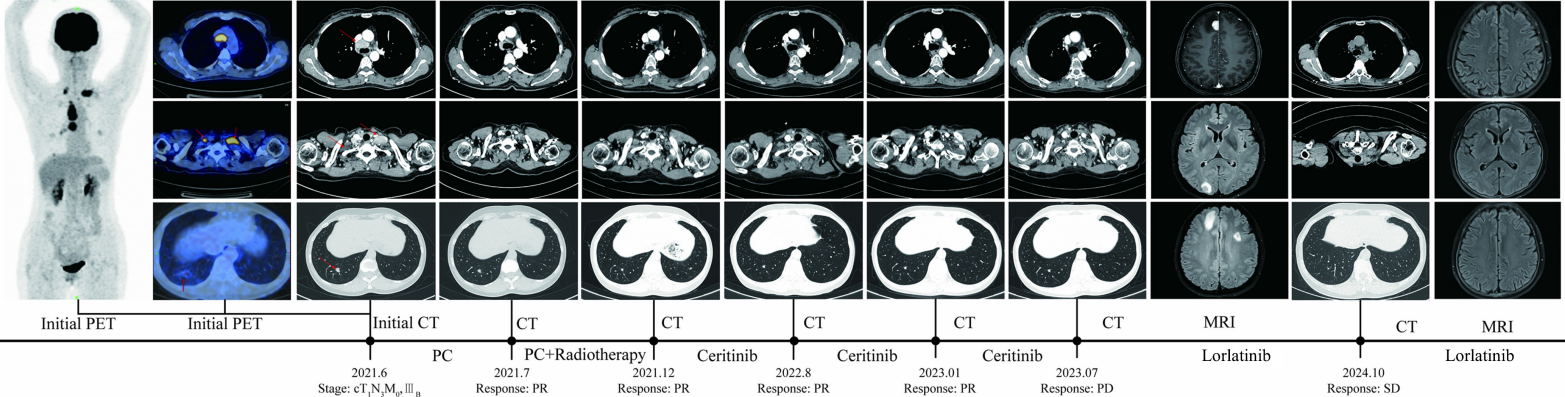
The initial PET/CT scans and CT scans before and after treatment of Case 1. PC: the first cycle of pemetrexed (500 mg/m^2^) and carboplatin (AUC: 5) chemotherapy; PC + Radiotherapy:pemetrexed (500 mg/m^2^) and carboplatin (AUC: 5) every 3 weeks for the following two cycles and pemetrexed (500 mg/m^2^) for the fourth cycle, with concurrent supraclavicular and mediastinal region radiotherapy (PTV:60Gy/30F and GTVnd:66Gy/30F). Ceritinib: 450 mg orally per day. Lorlatinib: 100 mg orally per day; CT, computed tomography; MRI, nuclear magnetic resonance imaging; PD, progressive disease; PET, positron emission tomography; PR, partial remission; SD, stable disease. The red arrows point to the primary lung cancer and metastatic lymph nodes.
